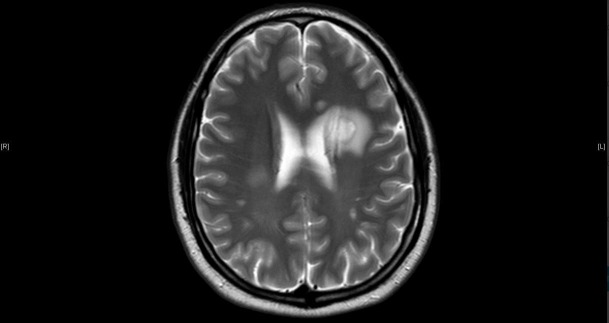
T2 MRI image showing the corresponding T2 enhancement of the aforementioned lesions.
